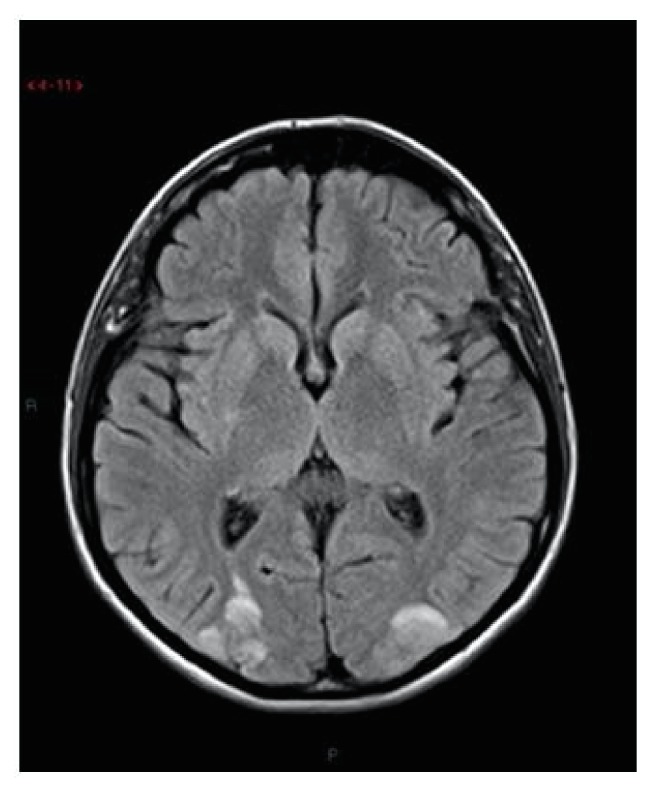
Axial FLAIR magnetic resonance images demonstrated bioccipital foci of high signal intensity involving the cortex and subcortical white matter.
